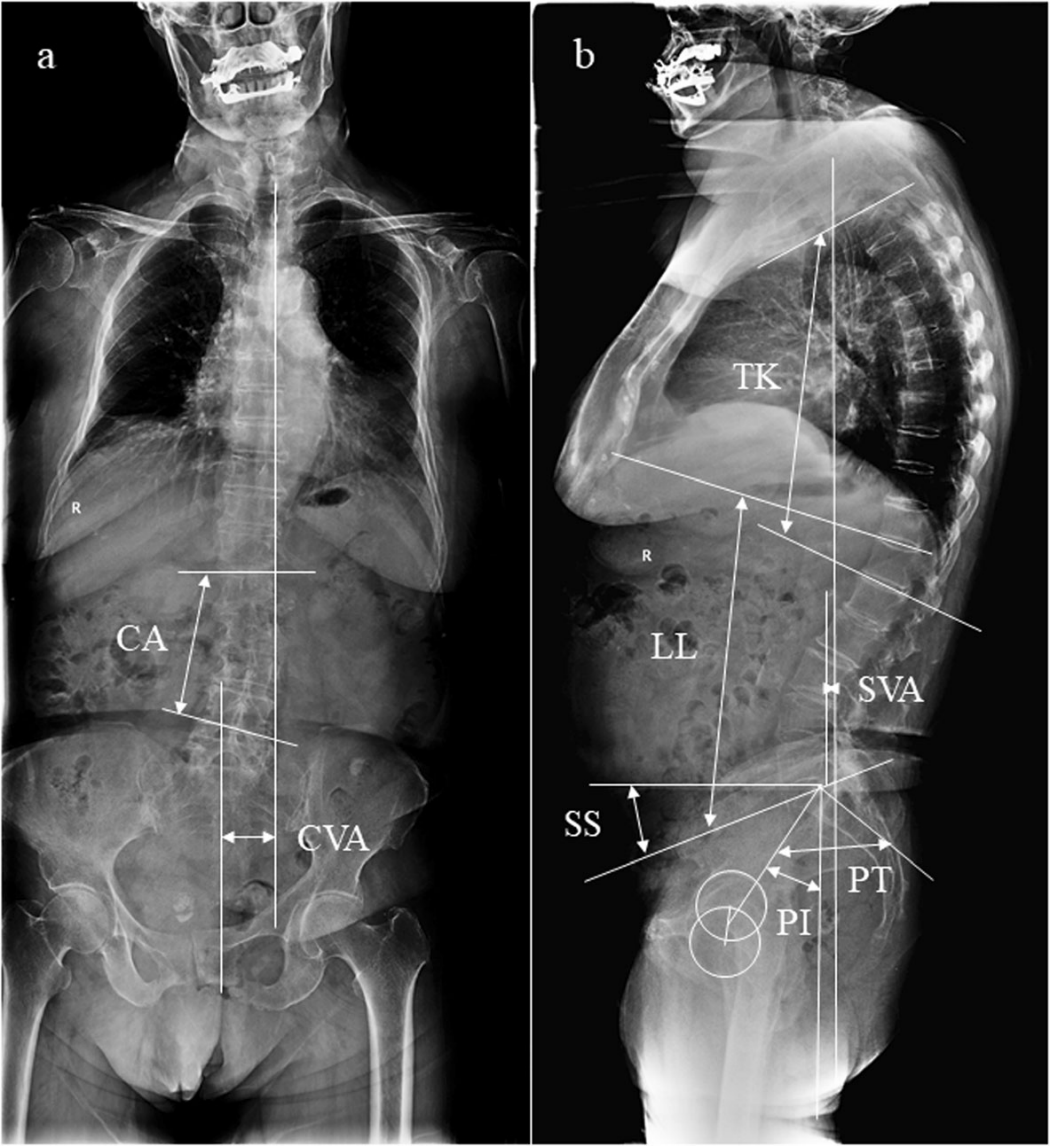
Radiological parameter measurement methods. a The coronal Cobb angle (CA) is measured from the superior end plate of the cephalad end vertebra and the inferior end plate of the caudal end vertebra on the coronal plane; the coronal vertical axis (CVA) is defined as the distance from a perpendicular line drawn from the superior end plate of S1 vertebral body to the C7 plumb line on the coronal plane. b The sagittal vertical axis (SVA) is defined as the distance from a perpendicular line drawn from the superior end plate of S1 vertebral body to the C7 plumb line on lateral radiographs; the thoracic kyphosis (TK) is measured from the upper end plate of T2 to the lower end plate of T12; the lumbar lordosis (LL) is measured from the upper end plate of T12 to the upper end plate of S1; the sacral slope (SS) is defined as the angle between the cranial sacral end plate and a horizontal line; the pelvic incidence (PI) is defined as the angle subtend by a line connecting the center of the femoral head to the center of the cephalad end plate of S1 and a perpendicular line from the upper end plate of S1; the pelvic tilt (PT) is measured as the angle between the vertical axis and the line through the midpoint of the sacral end plate to femoral heads axis
